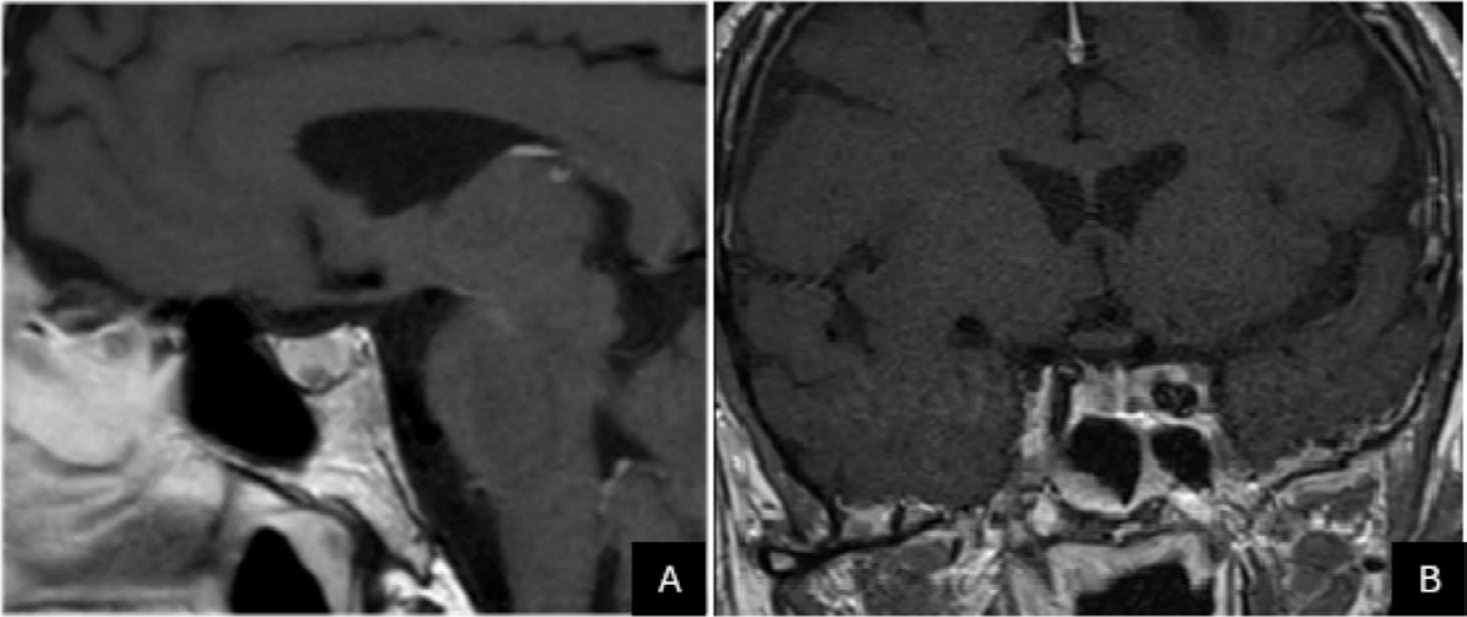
Pituitary MRI of patient revealed a 4 × 3 mm sized millimetric lesion that does not show enhancement in T1AG contrast‐enhanced series (cystic microadenoma with dense content?), sagittal (A), coronal (B).
